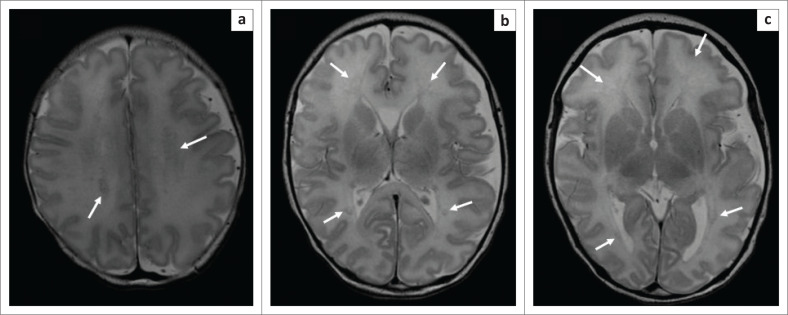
Axial T2-weighted MRI of the brain (repetition time msec/echo time msec, 3680/90, 3-mm section thickness) at the level of the high frontal lobes (a), basal ganglia (b) and peritrigonal white matter (c). The images show areas of T2 signal abnormality involving the corpus callosum and the bilateral supratentorial white matter, predominantly the bilateral frontal regions (solid white arrows on all images) with sparing of the thalami.
